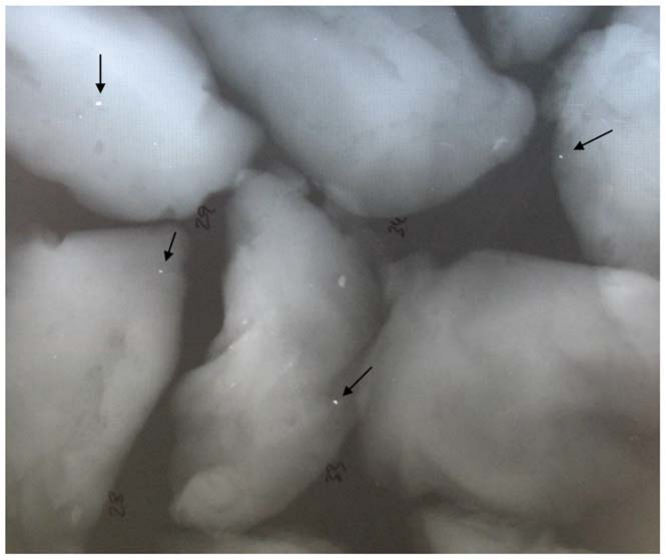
X-ray of breast muscle tissues from red-legged patridges containing small fragments of Pb left by the Pb shot pellets that were used as ammunition to hunt the birds. Fragments are generated as whole shot passes through tissue and fragments on impact.
